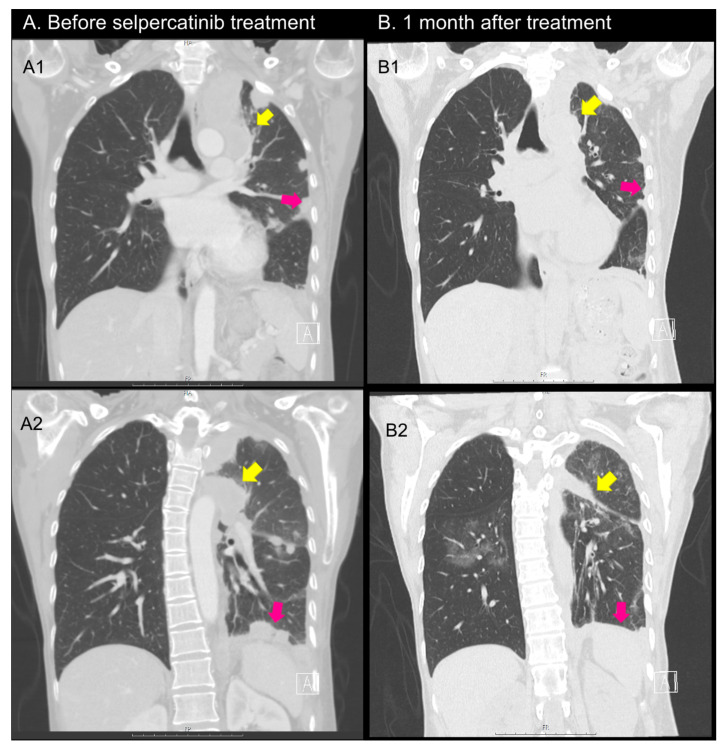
Before and at one month after selpercatinib treatment CT-scan of the patient in Case 4. Before treatment, there were metastatic lesions at hilar lesion, pleural, and lung parenchyme (A1,A2). After the treatment, all of them showed regression (B1,B2, yellow and purple arrows).
